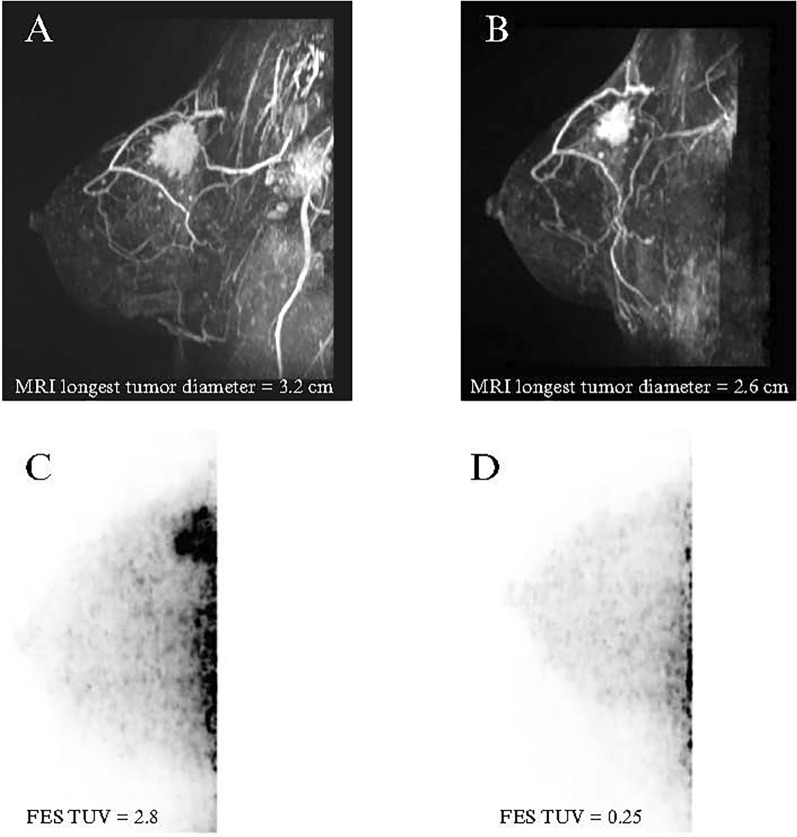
PID 17-images in a 32 year old patient with right breast invasive lobular carcinoma. A, B Sagittal maximum intensity projection post contrast T1-weighted subtracted 3D MRI scans show minimal change in enhancing tumor volume from (A) baseline to (B) 24 weeks after initiating neoadjuvant endocrine therapy with a SERM/SERD. C, D In contrast, sagittal maximum intensity projection dedicated breast PET images show significant decrease in 18F-fluoroestradiol uptake from (C) baseline to (D) 24 weeks after initiating neoadjuvant endocrine therapy with a SERM/SERD, consistent with ER blockade. SERM: selective estrogen receptor modulator, SERD: selective estrogen receptor degrader
